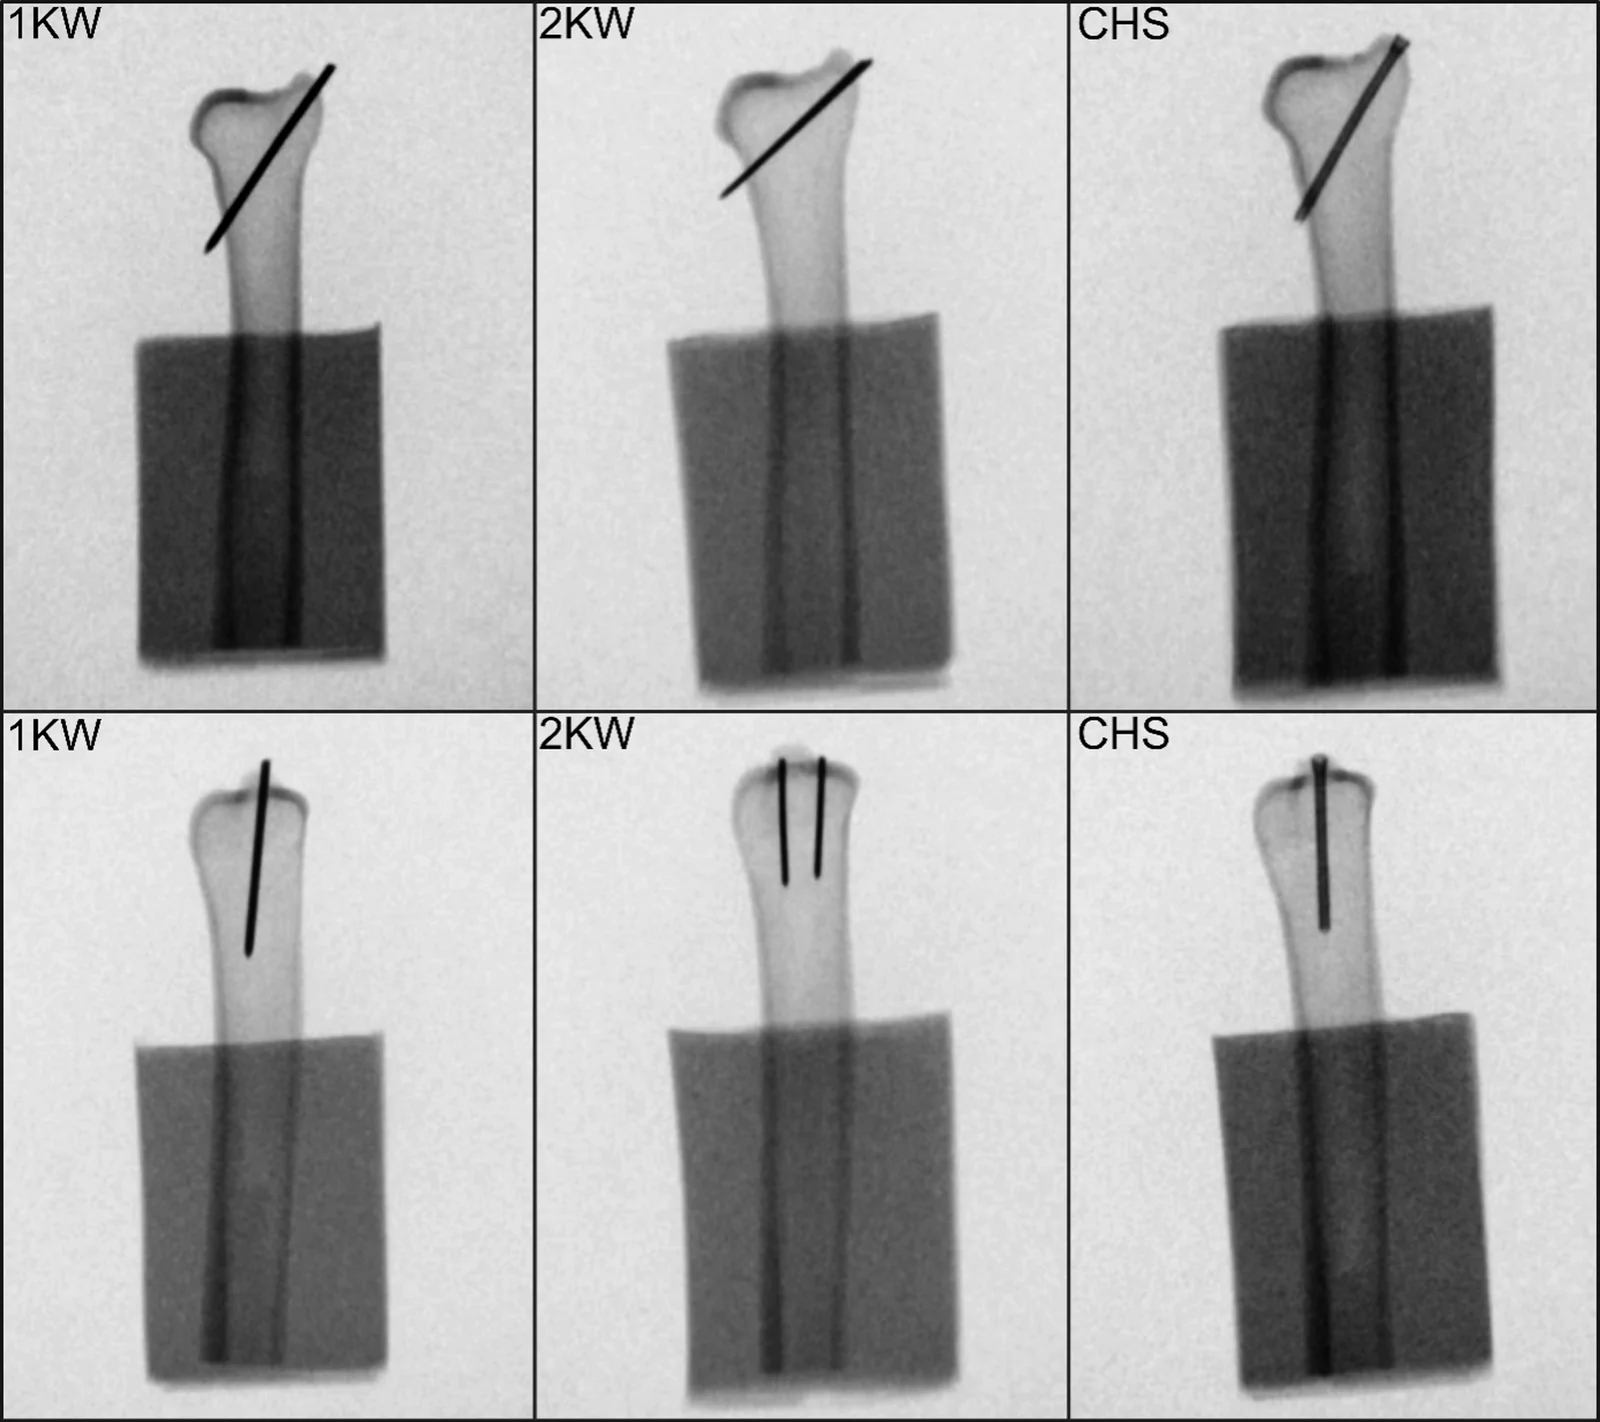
X-Rays of Sawbones after osteosynthesis of the performed osteotomy in ap-view (top row) and lateral-view (bottom row). 1KW = 1 K-Wire, 2KW = 2 K-Wires. CHS = Cannulated compression screw
